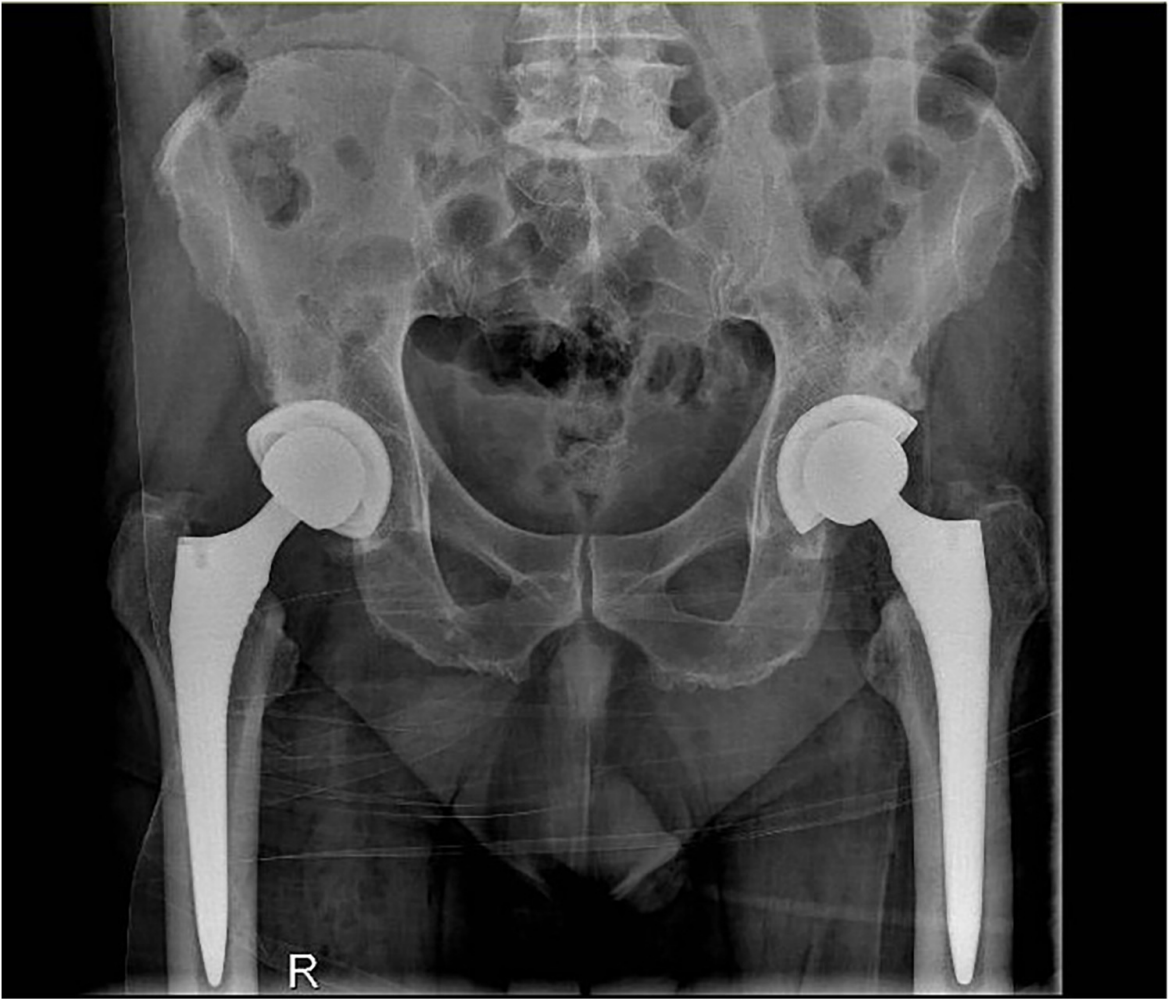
Postoperative radiography of bilateral total hip arthroplasty.
